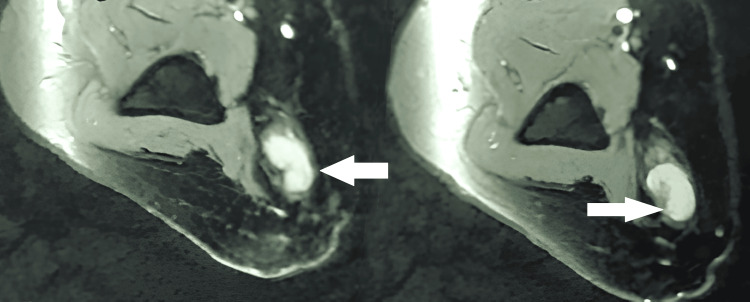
Magnetic resonance imaging (oblique-axial view) demonstrates a mass originating from the left ulnar nerve at the elbow
